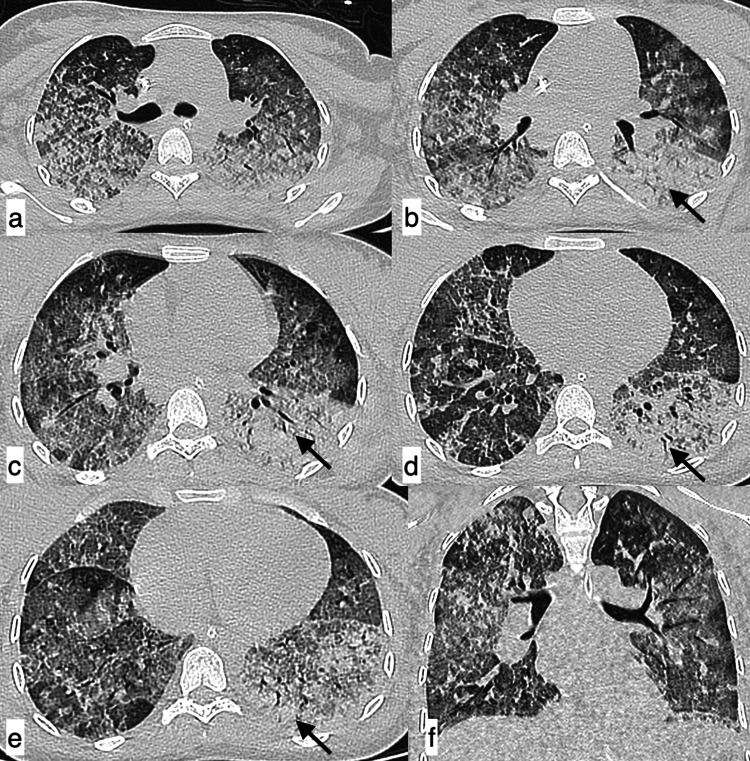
Diffuse alveolar haemorrhage with coexistent infection A 26-year-old female patient with SLE presented with haemoptysis, fever, and cough of two-day duration. Axial (a-e) and coronal (f) HRCT chest images in the lung window revealed extensive areas of ground-glass attenuation with associated interlobular septal thickening in both lung fields, with a large area of consolidation (black arrow) in the left lower lobe; imaging diagnosis of diffuse alveolar haemorrhage with coexistent infection was made, which was confirmed clinically. SLE: systemic lupus erythematosus; HRCT: high-resolution CT
